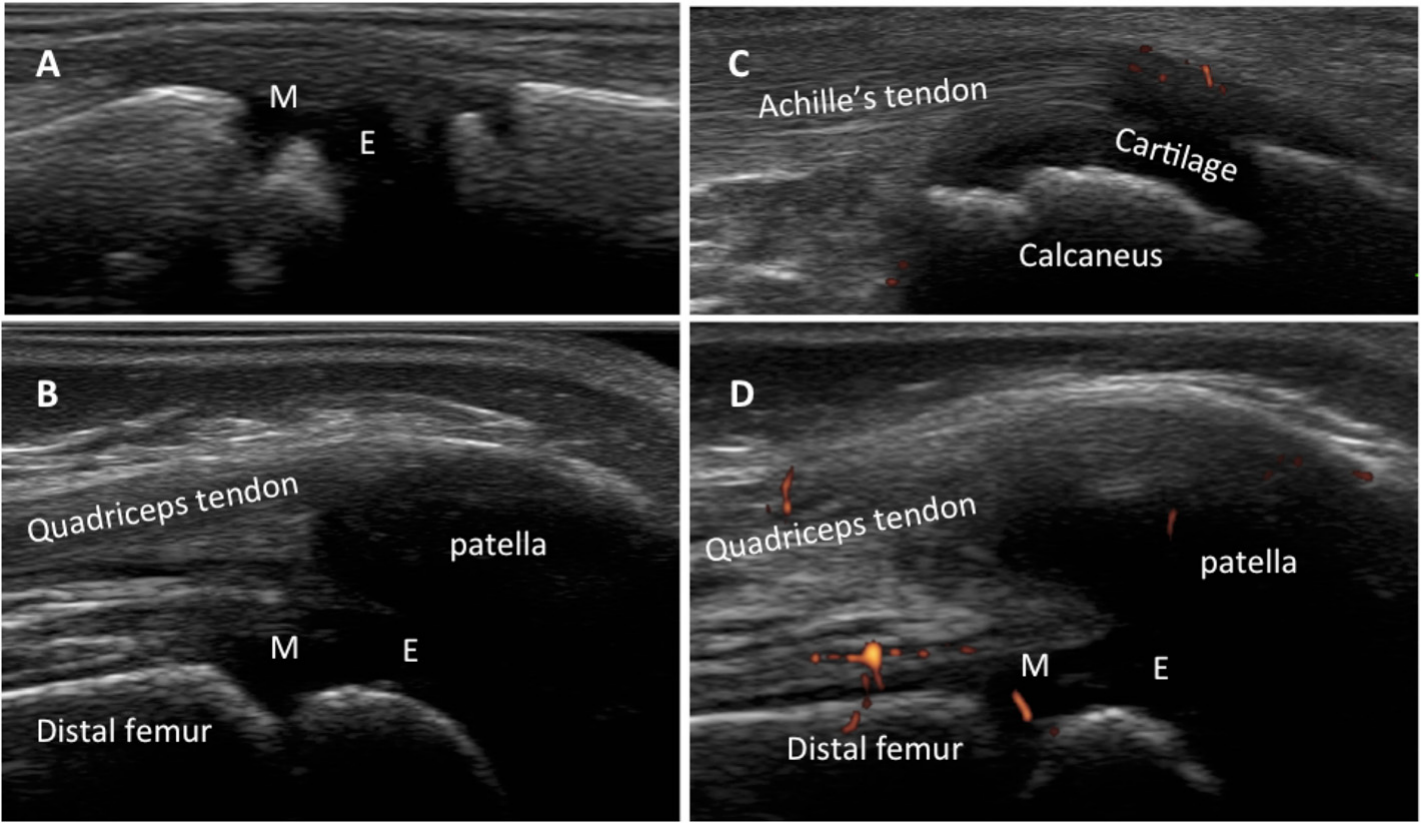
Metaphysis (M) look like erosions; epiphysis (E) and unossified bones are anechoic, like synovial effusion (a,b). Physiological vascularization at insertion of enthesis to the cartilage can be frequently detected, resembling enthesitis (c). Feeding vessels can be intraarticular or close to ossifying nuclei, and must not be considered as signs of active synovitis (d). a. Dorsal longitudinal scan of the II metacarpophalangeal joint in a 2 years old child, *grey-scale*. b. Longitudinal medial scan of the suprapatellar recess in a 2 years old child, *grey-scale*. c. Longitudinal scan of the Achilles tendon at insertion to the calcaneus in a 5 years old child, *power Doppler *
